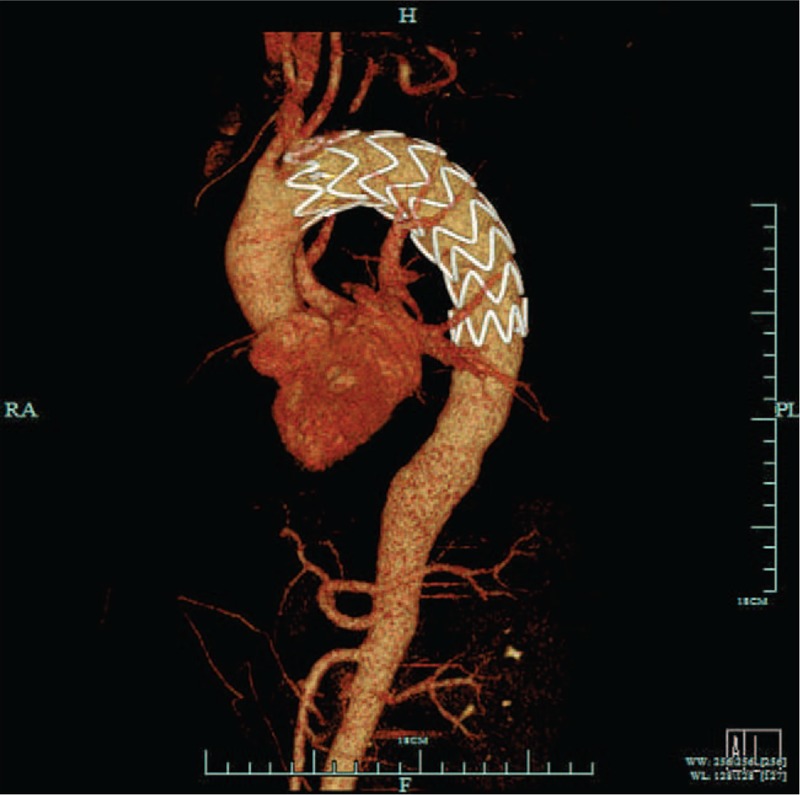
Thoracic aorta CTA of the patient after surgery. The aorta stent is displayed, which was found in the correct place. CTA = computed tomography angiography.
